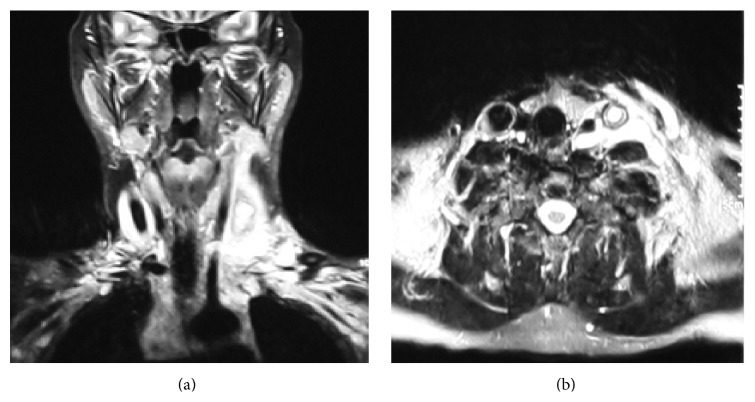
Magnetic resonance imaging of the left internal jugular vein thrombosis: (a) coronal plane, (b) axial plane.
